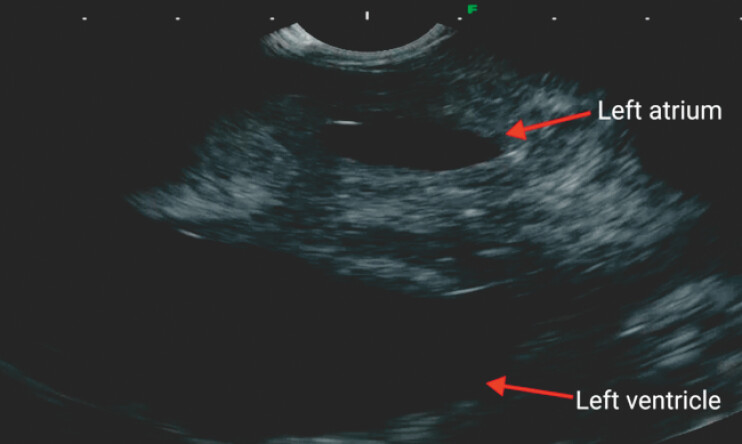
EUS demonstrated the left atrium and left ventricle, with the significant thickening of the left atrial wall. EUS, endoscopic ultrasound.
